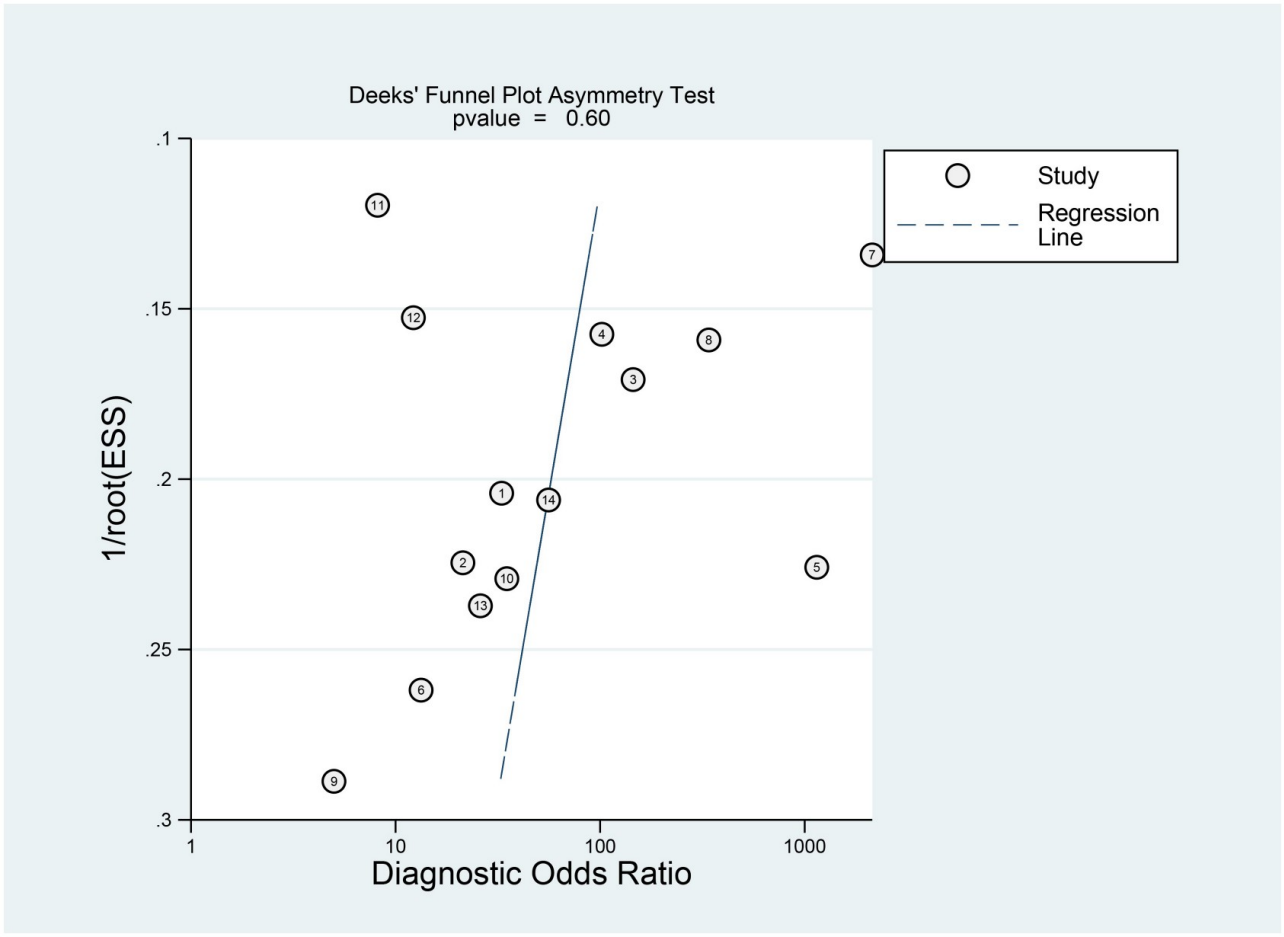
Funnel plot of the diagnostic value of PET/CT.
